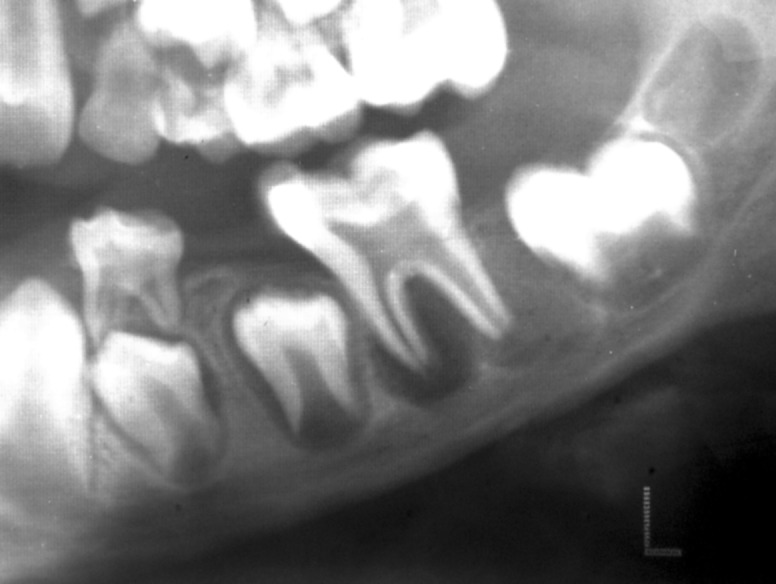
Preoperative radiograph revealing periapical
